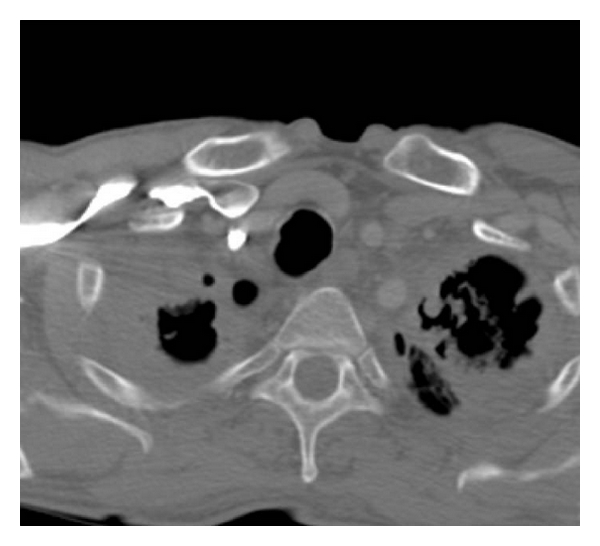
On a CT scan view of the upper lobes cavity lesions are demonstrated, with new shadows inside them, most probably due to aspergillus infection.
